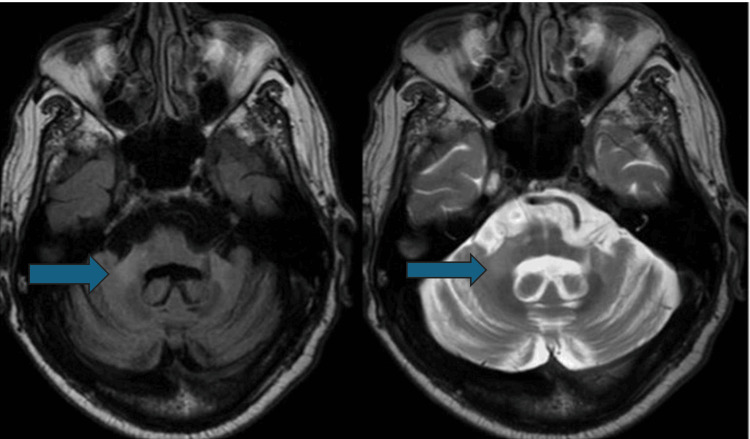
FLAIR and T2 axial images showing bilateral symmetrical hyperintensity of MCP (bright MCP sign) in MSA-C. Diffuse cerebellar atrophy is also noted. Arrows in these images are pointing toward the hyperintensity of the bilateral MCP (bright MCP sign). FLAIR = fluid-attenuated inversion recovery; MCP = middle cerebellar peduncle; MSA-C = multiple system atrophy-cerebellar
